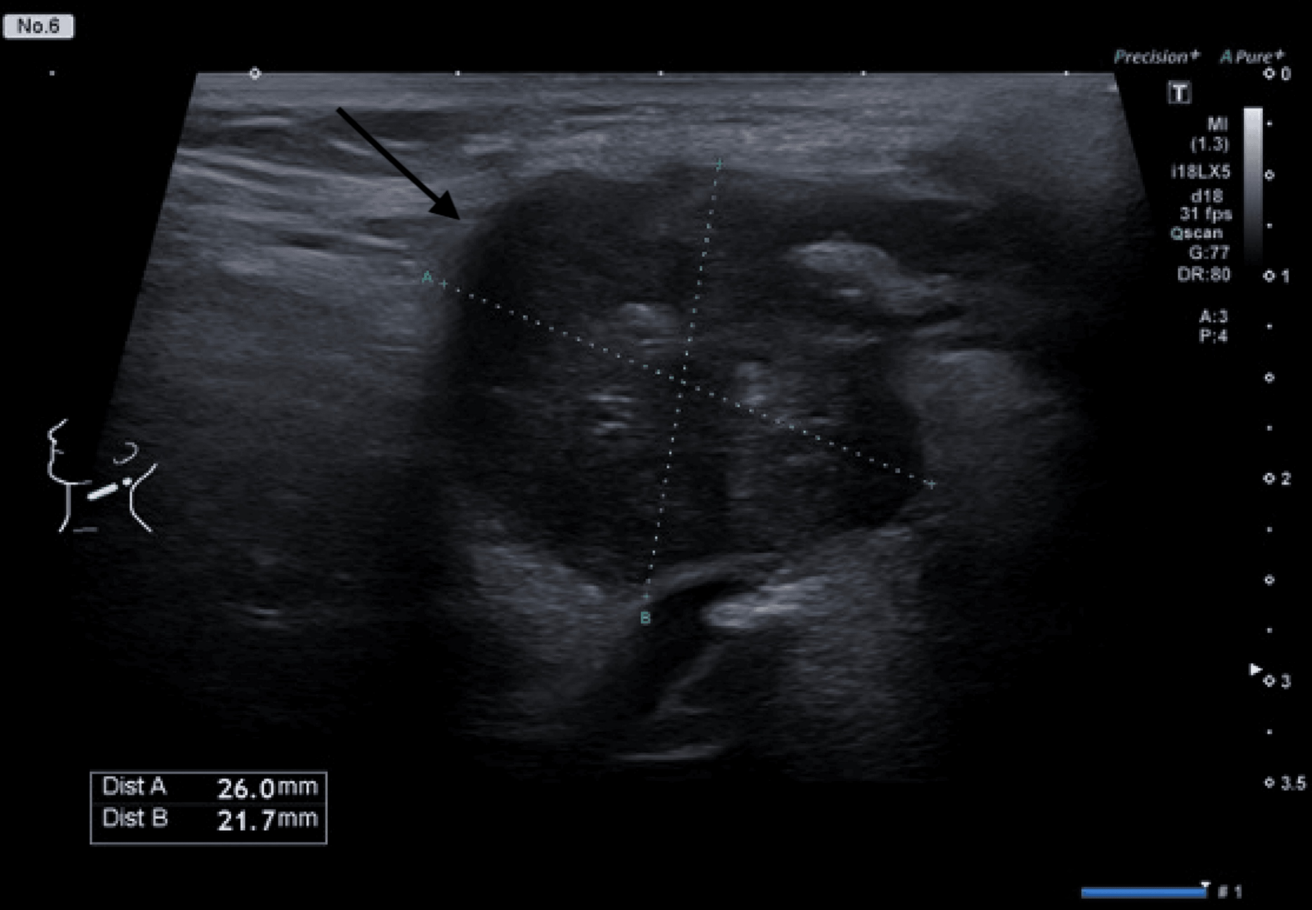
Ultrasound showing 2.6 x 2.2 x 2.0 cm (arrow) affected lymph node.
